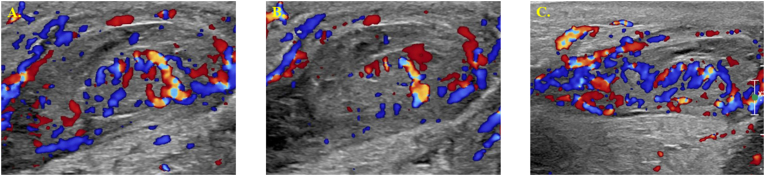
Ultrasound results on Initial ED Presentation. (A) Right Testicle, (B) Right Epididymal Head, (C) Right Inguinal Canal
